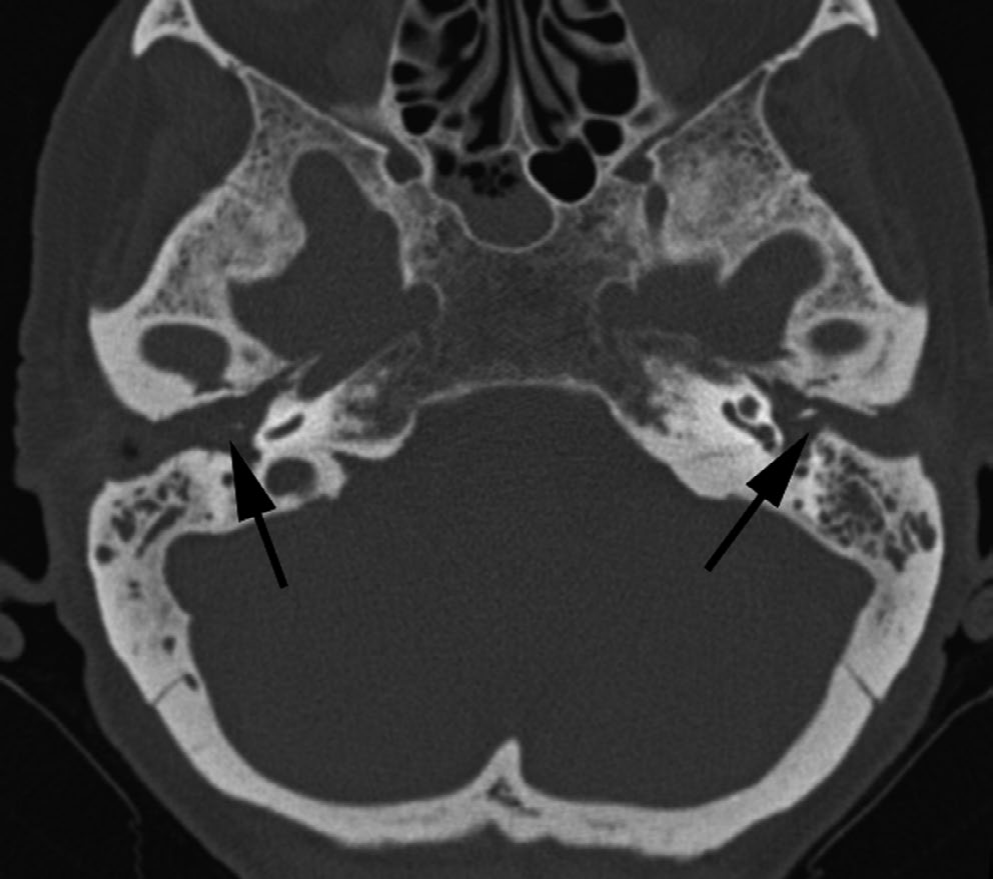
Axial computed tomography (CT) scans of the middle ear. Black arrows showing a bilateral EAC stenosis and isodense tissue occupying the whole middle ear, bilaterally
